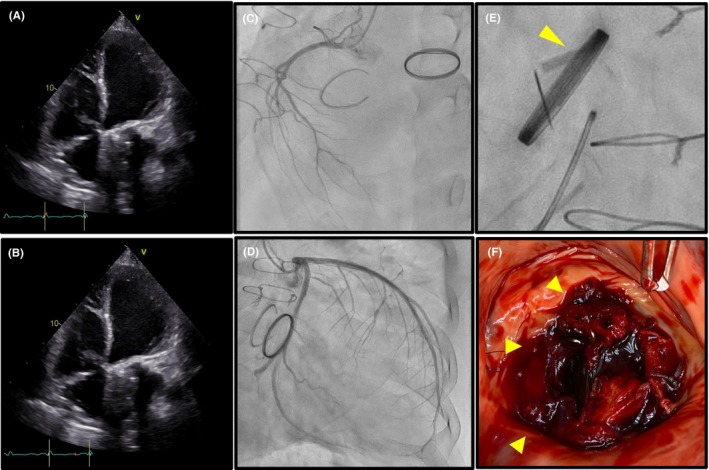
(A, B) Transthoracic echocardiography shows severe biventricular dysfunction (A: end‐diastolic, B: end‐systolic). (C, D) Coronary angiography reveals normal coronary flow (C: right coronary artery, D: left coronary artery). (E) The lateral leaflet of the mechanical valve is dysfunctional and nonmobile (yellow arrowhead). (F) Autopsy findings reveal an organized thrombus stacked in the lateral leaflet (yellow arrowhead), while the mobility of the medial leaflet is unaffected.
